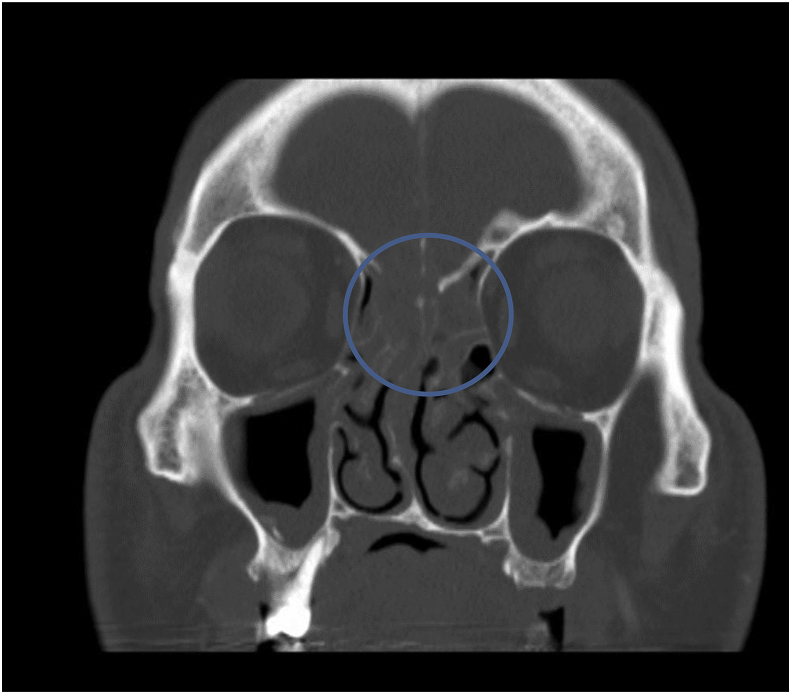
Coronal CT scan of the sinuses showing frontal ethmoidal recesses obstructed bilaterally. Moderate opacification of the ethmoid air cells bilaterally is present. Right sphenoid sinus is hypoplastic and moderately opacified. The right sphenoethmoidal recess is obstructed. Complete obstruction of the ostiomeatal units is present bilaterally due to mucosal thickening.
